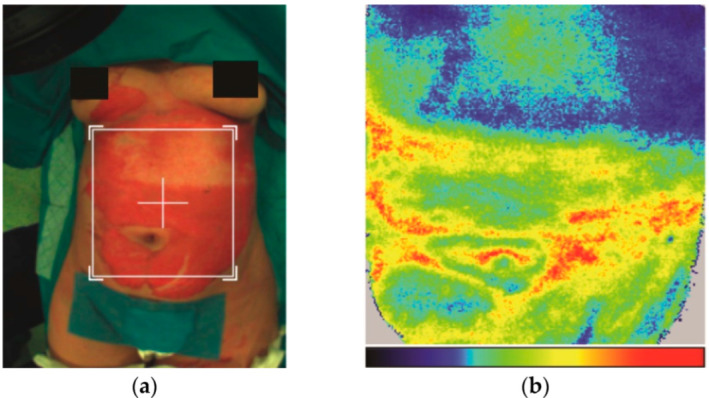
(a,b) Clinical appearance and laser Doppler imaging of the same area before enzymatic debridement with Nexobrid^®^. Images from the authors’ collection.
